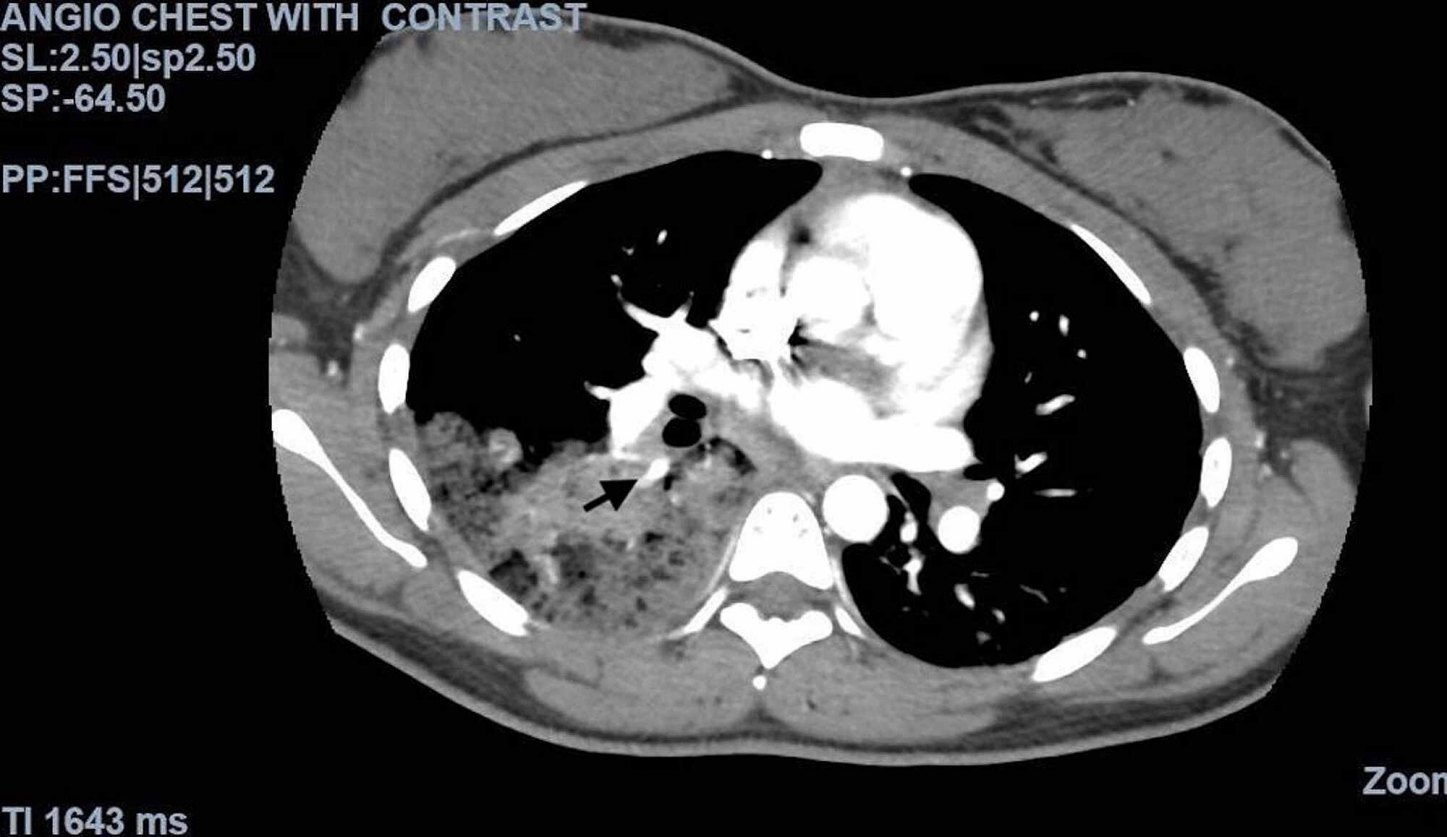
CT angiogram of the chest with IV contrast The image shows acute pulmonary emboli involving the right lower lobe CT: computed tomography; IV: intravenous
